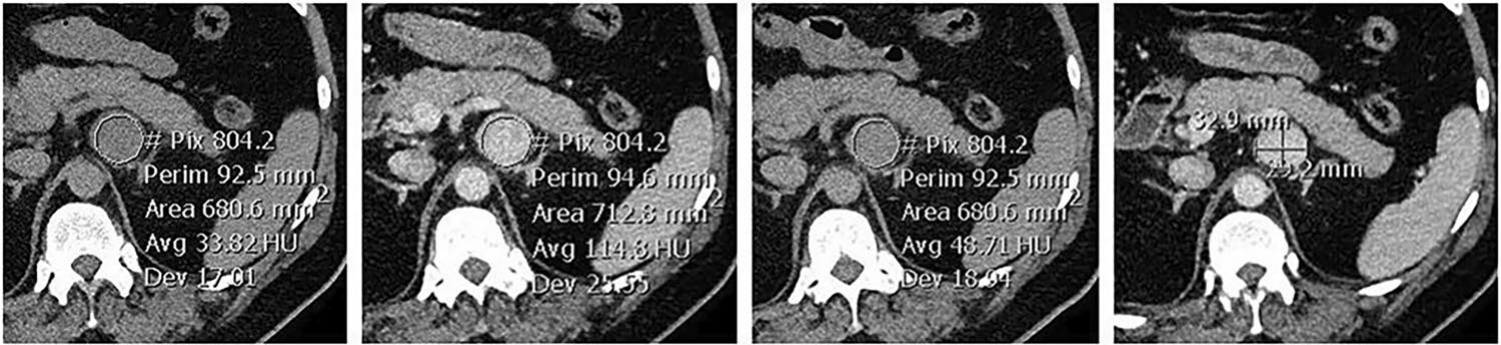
Adrenal washout computed tomography with axial slices demonstrating a left adrenal nodule with mean attenuation measurements on non-contrast enhanced (left), portal venous (middle left) and delayed (middle right) acquisitions. Long and short-axis measurements (right)
